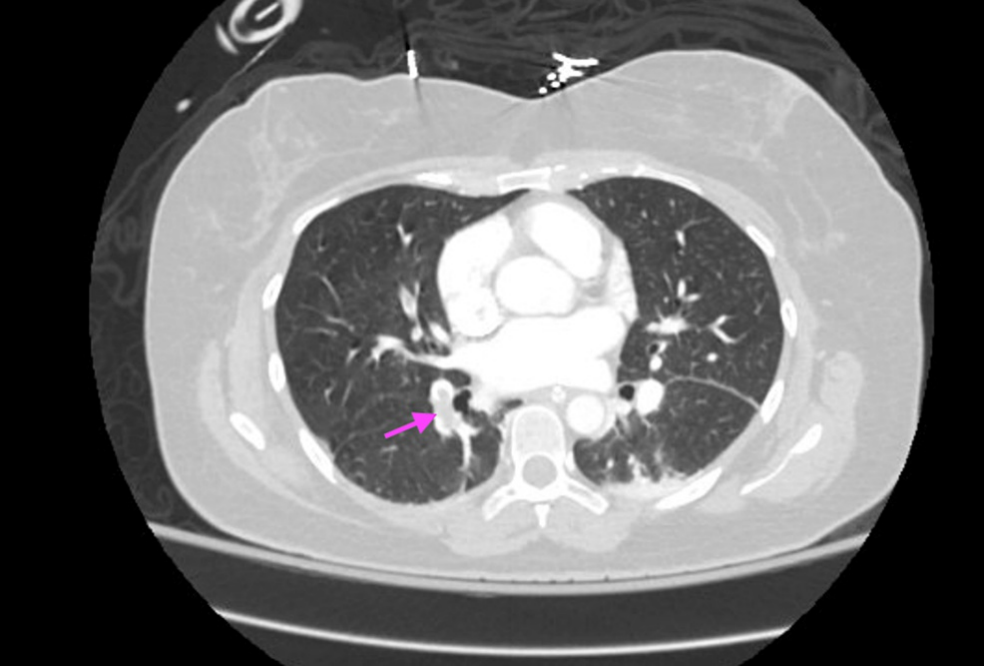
Incompletely occlusive pulmonary emboli involving the right-sided pulmonary arteries seen on CT angiogram of the lungs in Case 3
